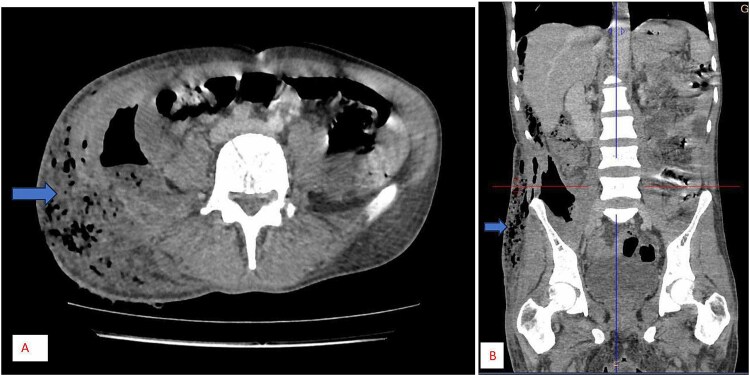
Preoperative contrast-enhanced CT abdomen and pelvic images axial (A) and coronal (B) showing extensive right lumbar necrotizing soft tissue infection with multiple air foci and surrounding inflammatory stranding (arrows).
